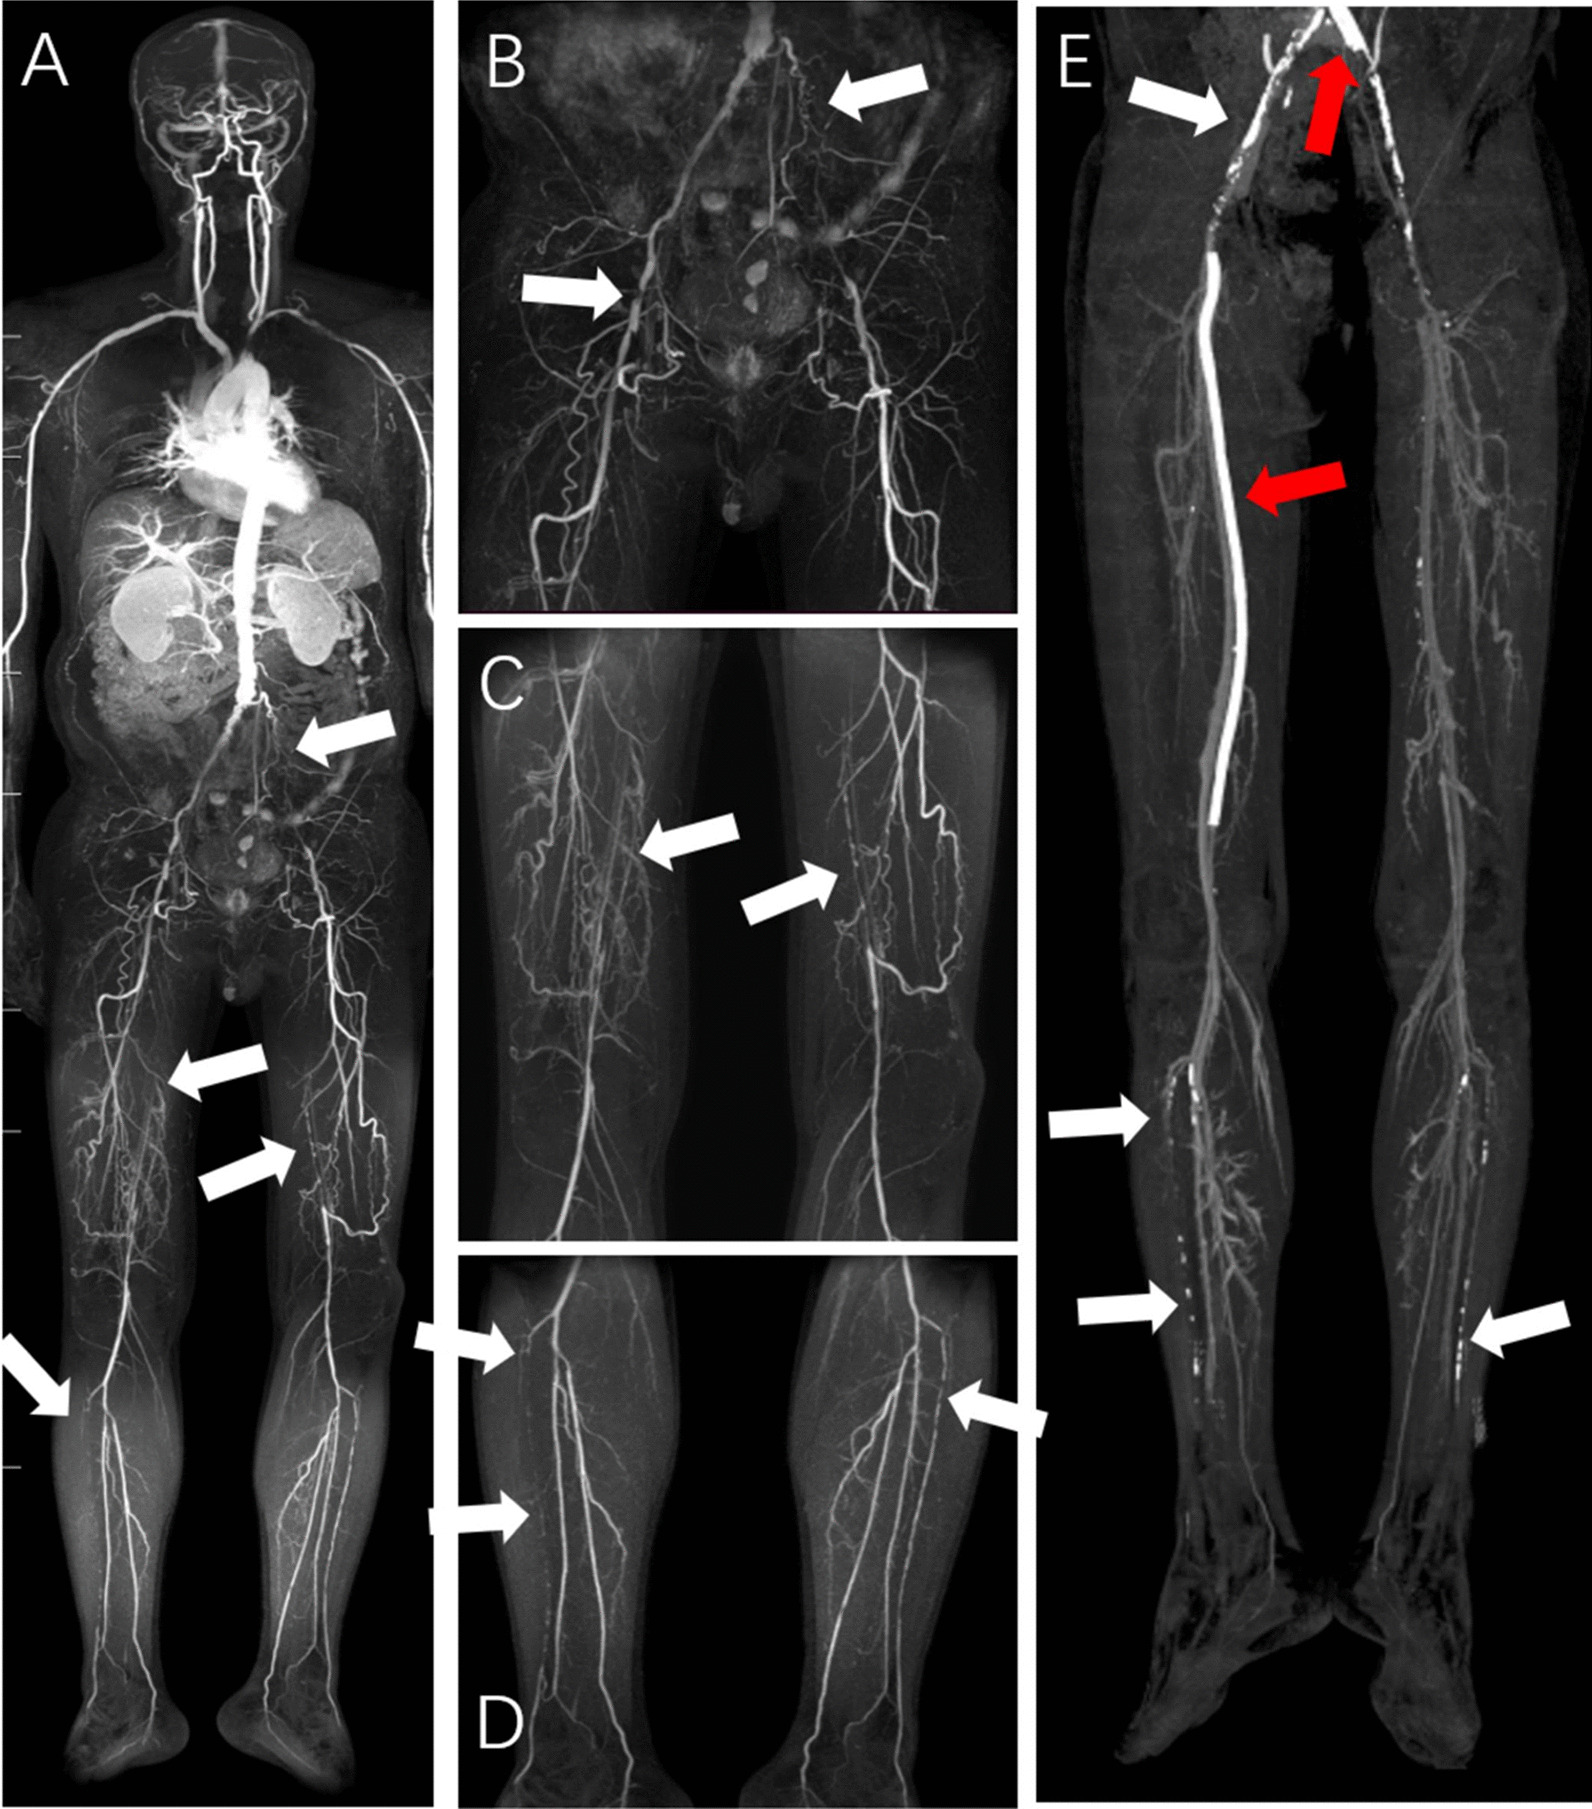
A male patient, 62 years old, had arteriosclerosis of lower extremities for several years. Whole-body subtractionless CS-WBMRA MIP (A–D) showed different grades of stenoses (white arrows). Three days after CS-WBMRA examination, the patient underwent stent placement surgery involving the left common iliac artery and the right superficial femoral artery. Corresponding CTA of the lower extremity (E) depicting the stents (red arrows) and CS-WBMRA findings clearly (white arrows)
